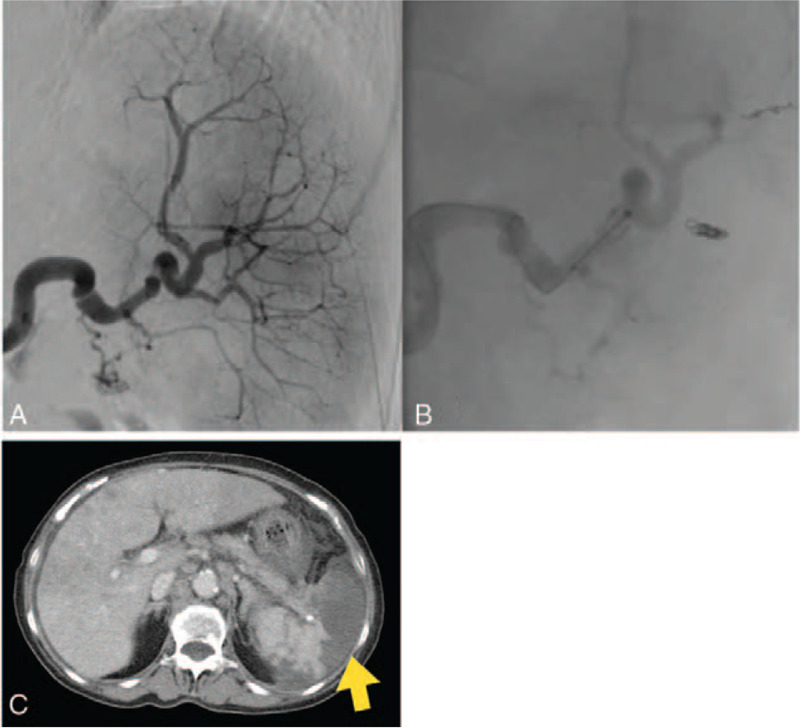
Selective arteriogram shows appearance before embolization. Post-PSE angiographic image showing 60% embolization using embospheres and microcoils performed in case 2. (C) Contrast-enhanced computed tomography scan acquired during portal phase showing splenomegaly with a large unenhanced area of the liquid (arrow) representing splenic necrosis. Approximately 60% of splenic parenchyma is ischemic.
